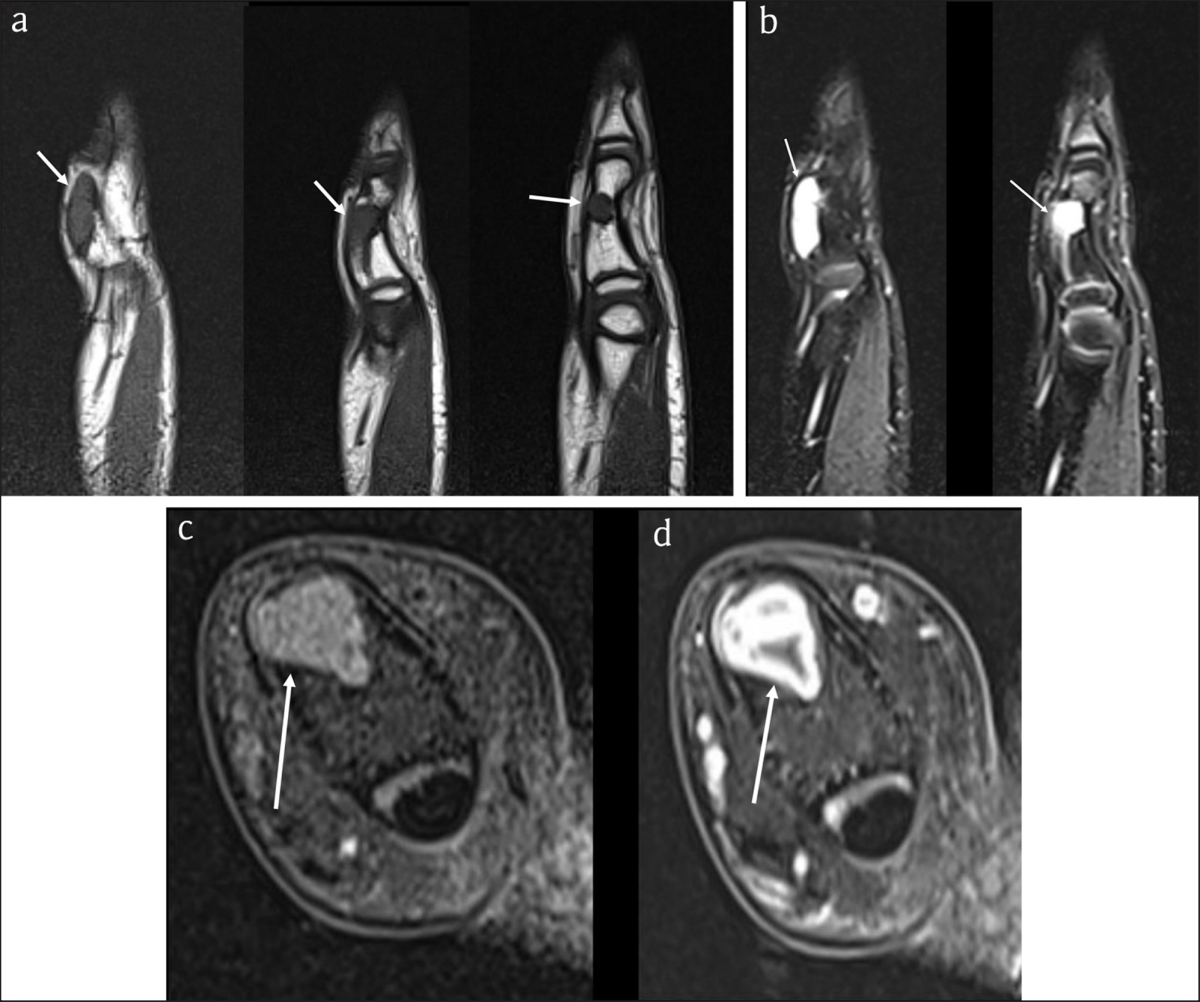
Case 1 – MRI. Sagittal T1-WI (3 adjacent slices) (a). Sagittal fat-suppressed (FS) T2-WI (2 adjacent slices) (b). Axial FS T1-WI (c). Axial FS T1-WI after intravenous administration of gadolinium contrast (d). The lesion is isointense to muscle on T1-WI (arrows in a) and hyperintense on FS T2-WI (arrows in b) and enhances peripherally, in keeping with chondroid matrix (arrow in d).
